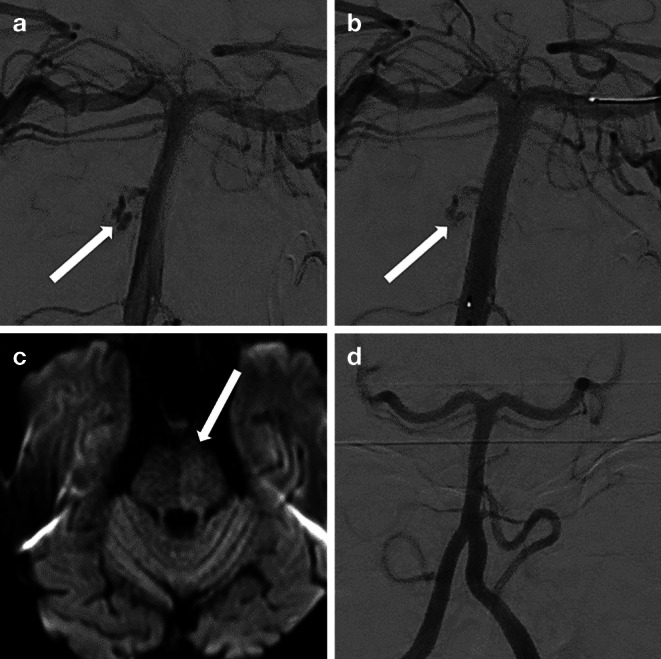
DSA of the basilar artery ap view (a) showing a possible active bleeding from a ruptured BAPA (patient #3). The patient suffered from two episodes of clinical worsening in the 2 h before the DSA. DSA ap view following implantation of the FD (b) with reduction of the contrast extravasation. Axial DW MR image (c) showing an increased signal intensity in the left paramedian area of the pons due to acute perforator artery occlusion. 6‑month follow-up DSA (d) showing complete occlusion (O’Kelly-Marotta occlusion score D) of the BAPA
